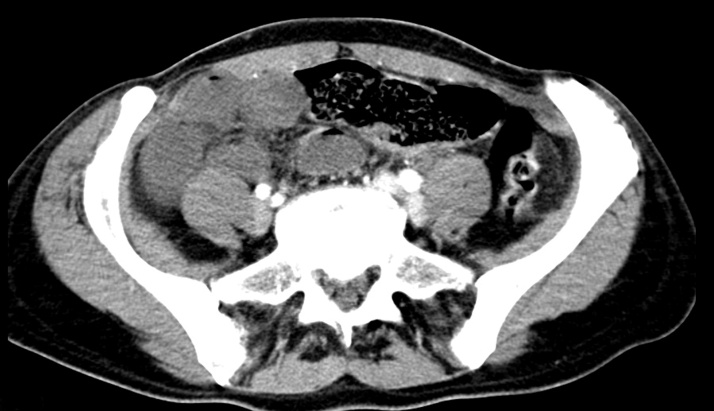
CT scan of the abdomen: conglutinated ileal loops in the right iliac fossa.
